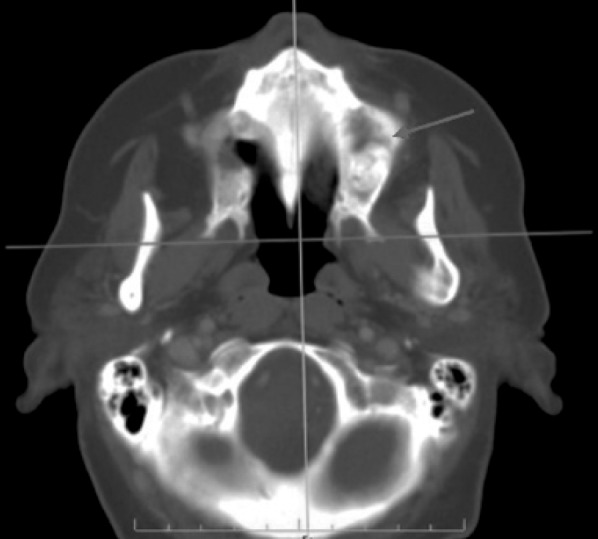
CT image demonstrating a large bone sequestrum in the affected area (red arrow).
